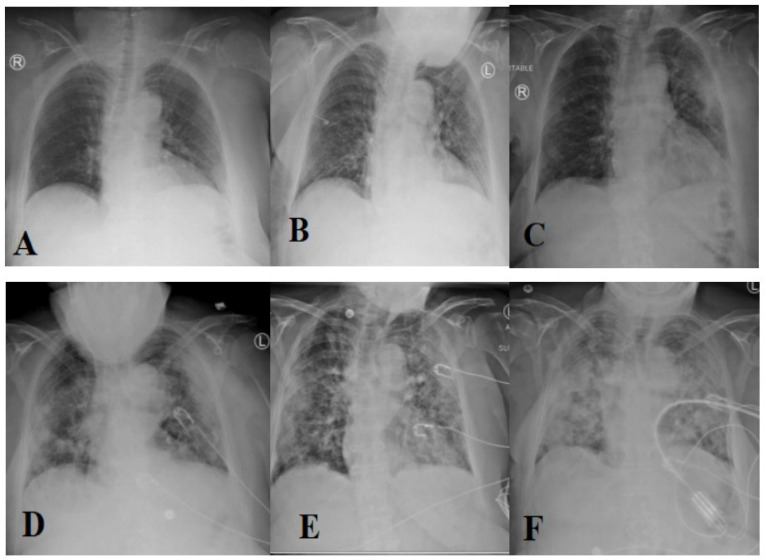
Evolution of an X-ray image for a COVID-19 patient over days (1 (A), 3 (B), 6 (C), 7 (D), 8 (E), and 10 (F), respectively) [11] (reproduced with permission).
